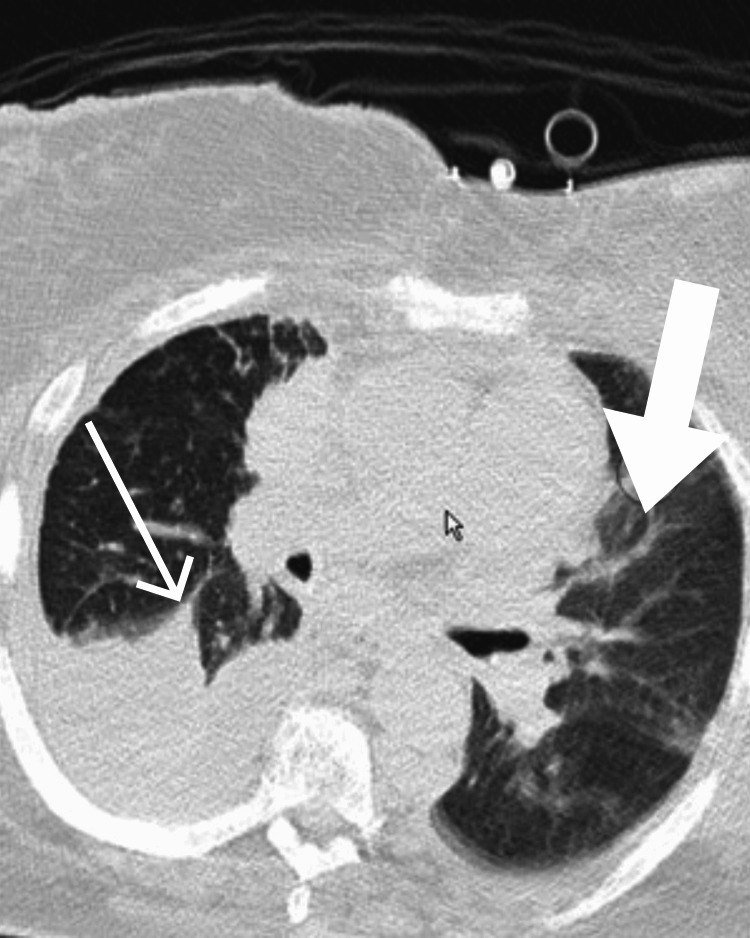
Computed tomography image showing left-loculated pleural effusion (large arrow) and right-sided pleural effusion (thin arrow).
